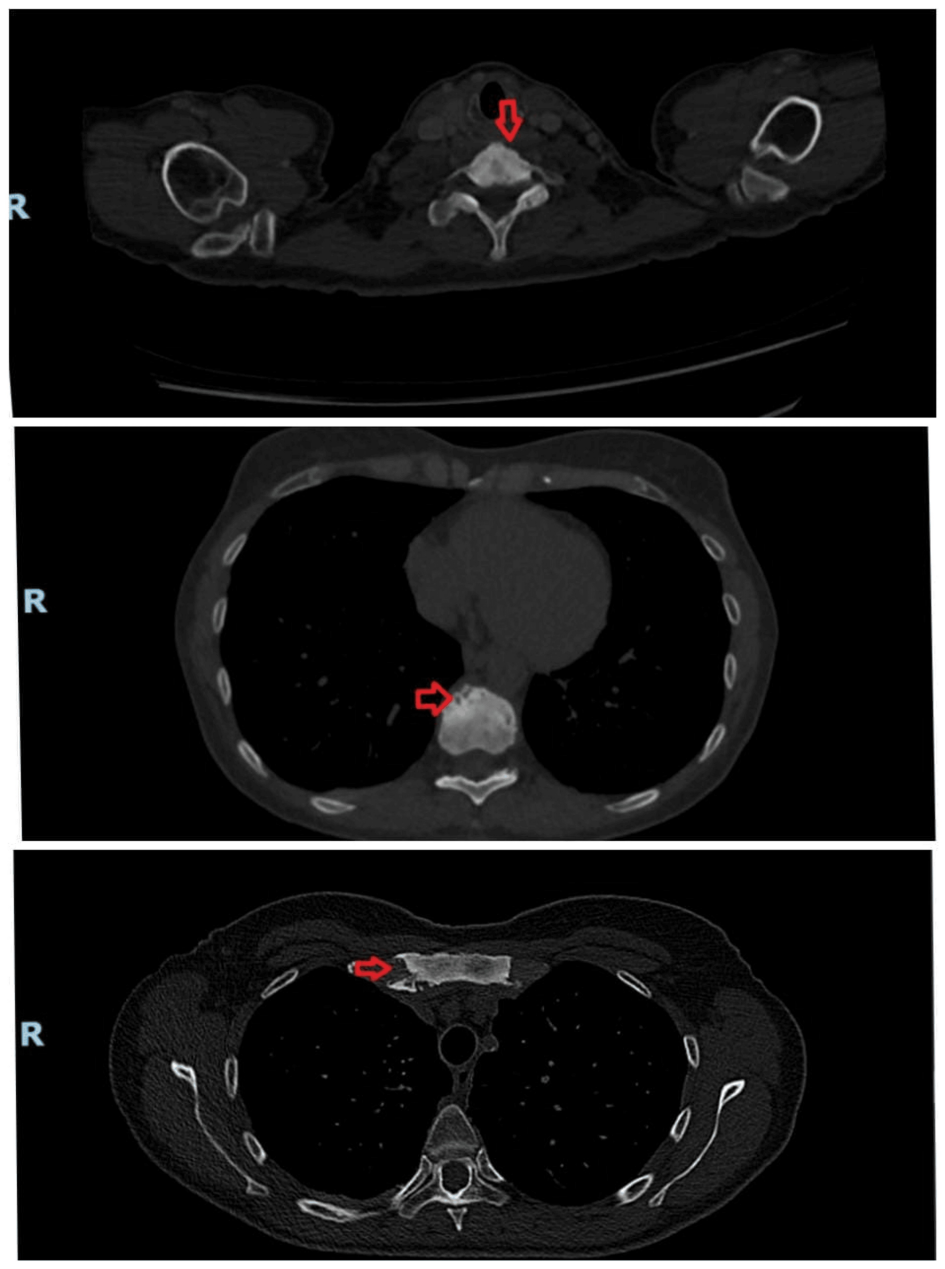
Axial CT scan in the bone window shows multiple sclerotic lesions in the vertebrae (cervical and thoracic) and in the manubrium
